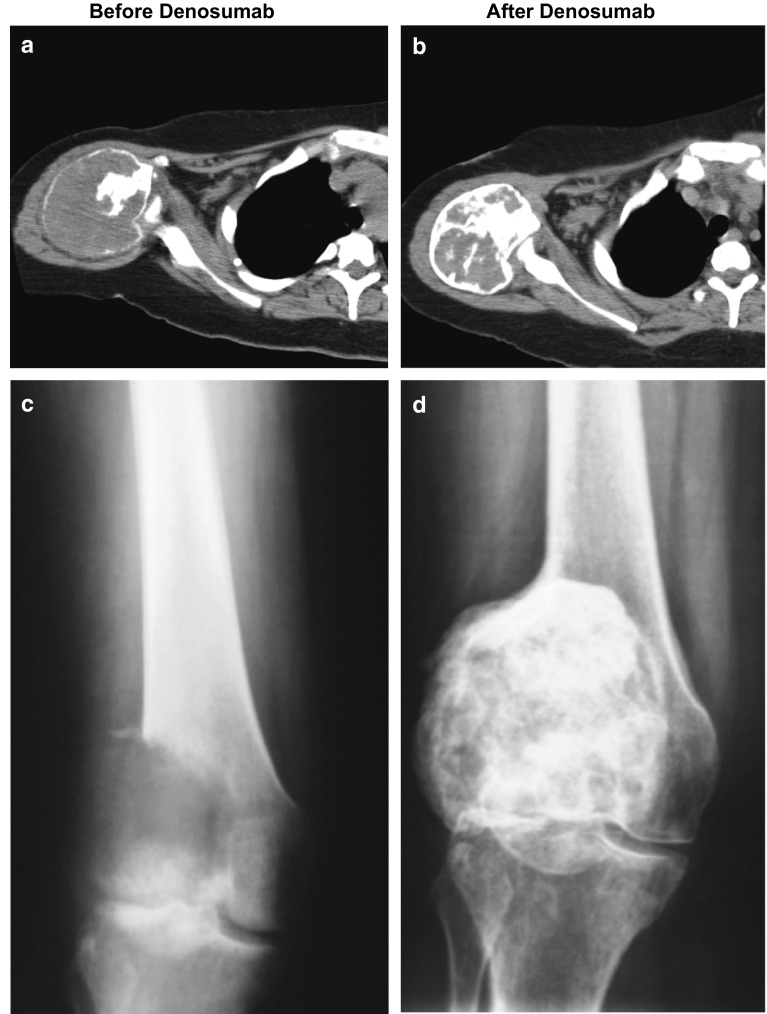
Example of radiographic images of giant cell tumor of bone of the proximal humerus and distal femur before (a, c) and after (b, d) denosumab therapy. The initial lesions were expansile with a thin peripheral calcified shell and primarily soft tissue density centrally (a) and showed extensive soft tissue displacement with progression following radiotherapy 2 years previously (c). After 4 months of treatment with denosumab, the peripheral calcification was thicker, the central lesion more heavily mineralized, and the overall size was slightly decreased (b, d)
